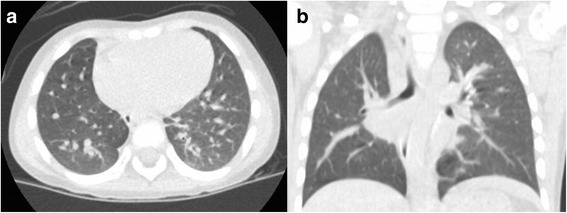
One-year postoperative computed tomography scan obtained from Patient 2. Lungs were well expanded with no residual cystic mass (a. axial, b. coronal)
